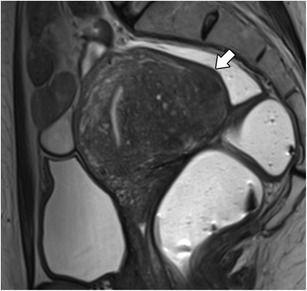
Adenomyoma: Sagittal T2-weighted image; circumscribed intra-myometrial hypointense mass with ill-defined margins and minimal mass effect with high-signal foci (white arrow)
